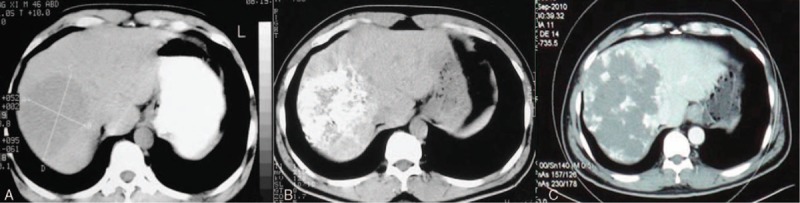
Change of liver hemangioma after TAE. (A) CT scan before TAE; (B) CT scan in 3 months after TAE, which showed the shrink of the tumor; (C) CT scan in 5 years after TAE, which showed the enlargement of the tumor. CT = computed tomography, TAE = transcatheter arterial embolization.
